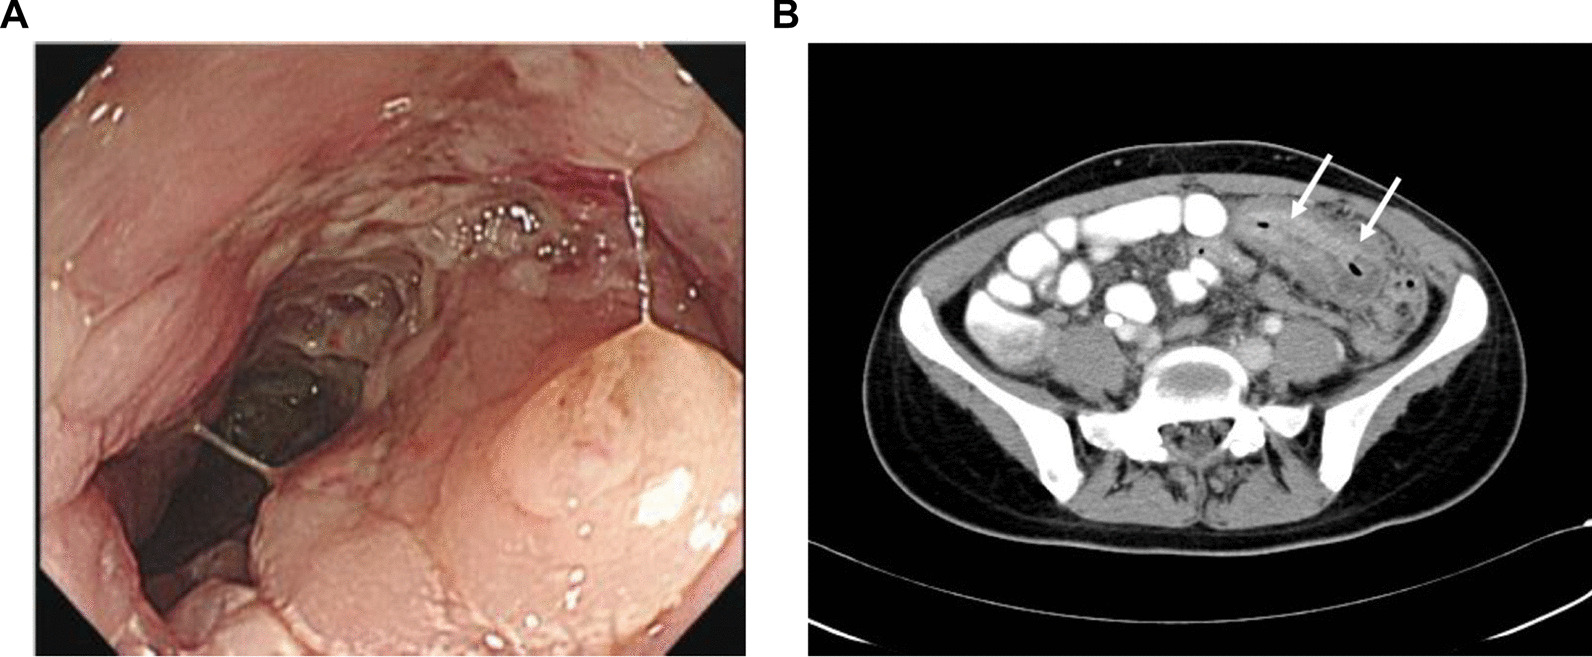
Typical endoscopic and CT image of SRCC. A The colonoscopy revealed edematous fragile mucosa with ulcer that led to lumen stenosis at transverse colon. B Abdominal computer tomography (CT) showed segmental wall-thickening of transverse colon with increased enhancement and prominent adjacent fat-stranding (arrow)
